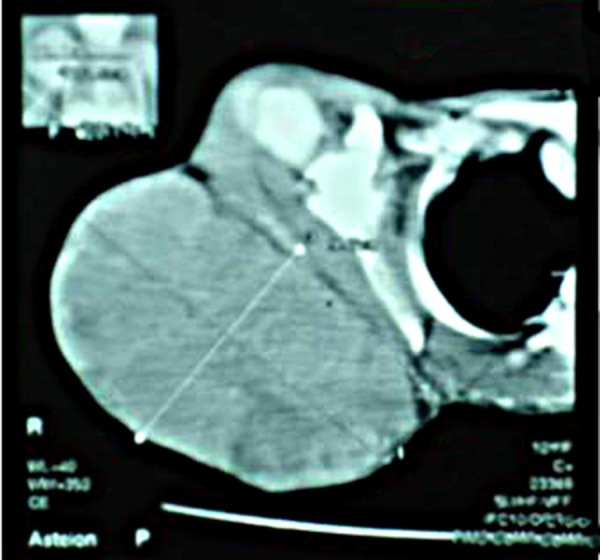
Figure 3: CT scan showing soft tissue tumour
